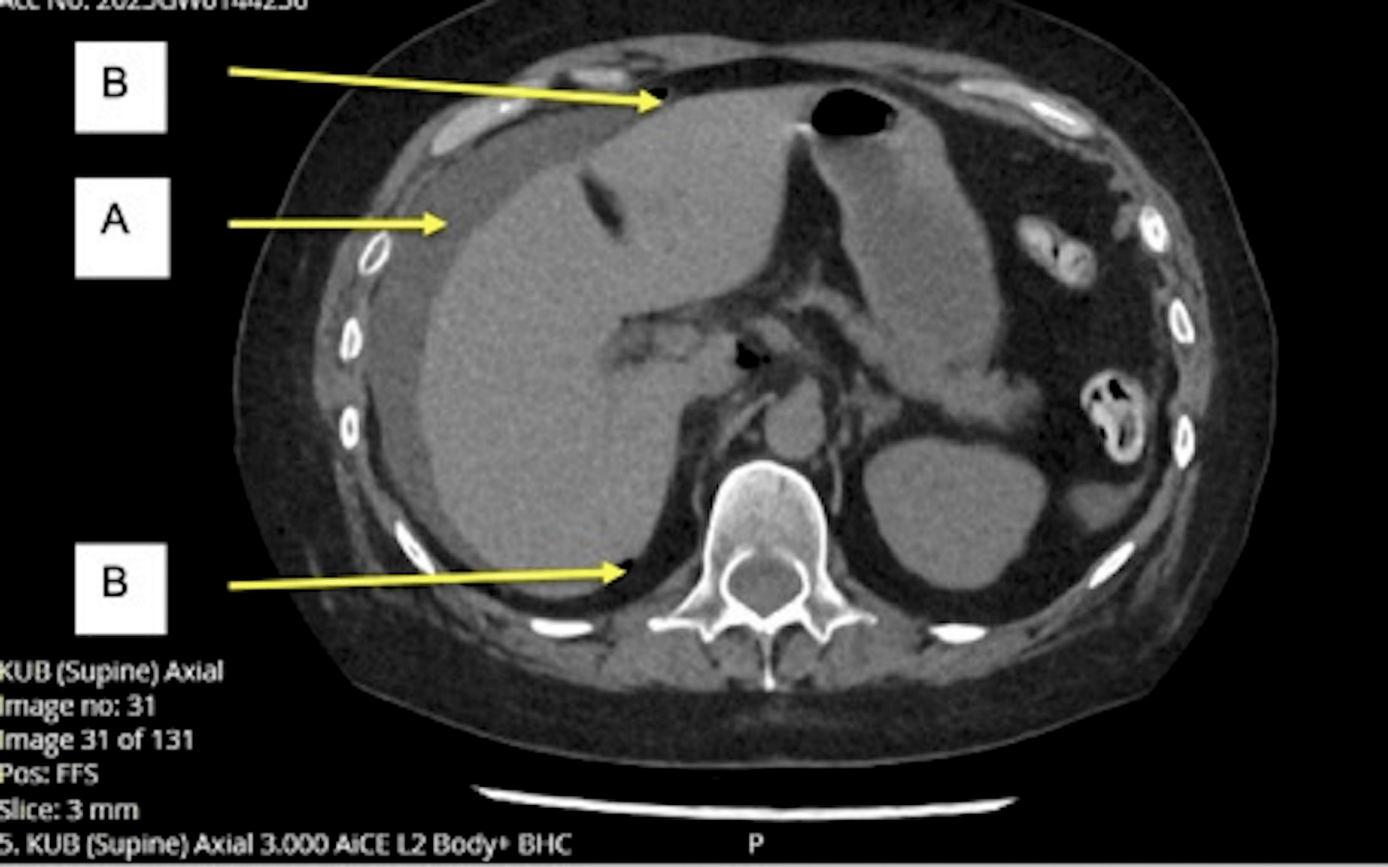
Axial slice of CT KUB demonstrating a moderate volume of free intraperitoneal fluid (A) and locules of extraluminal air (B) in the upper abdomen KUB, kidneys, ureters, and bladder
